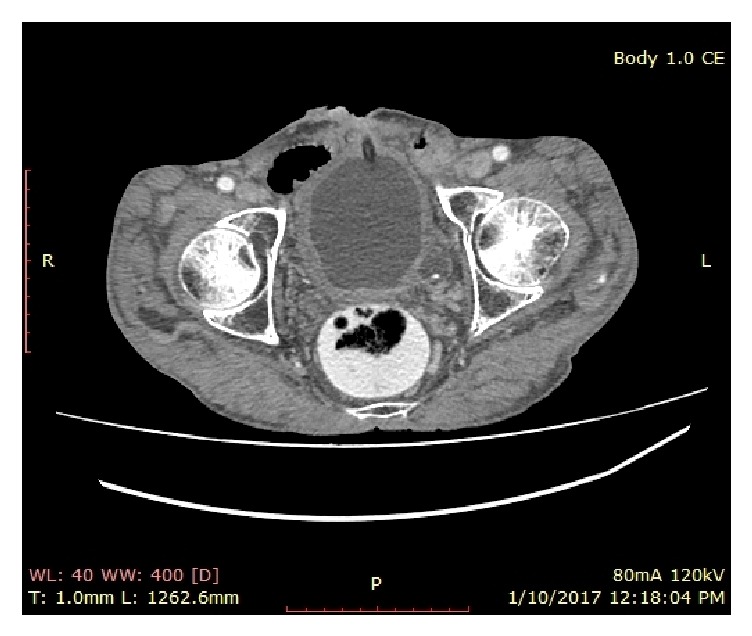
A contrast enhanced computed tomography scan image of suprapubic cystostomy site growth, transverse section.
